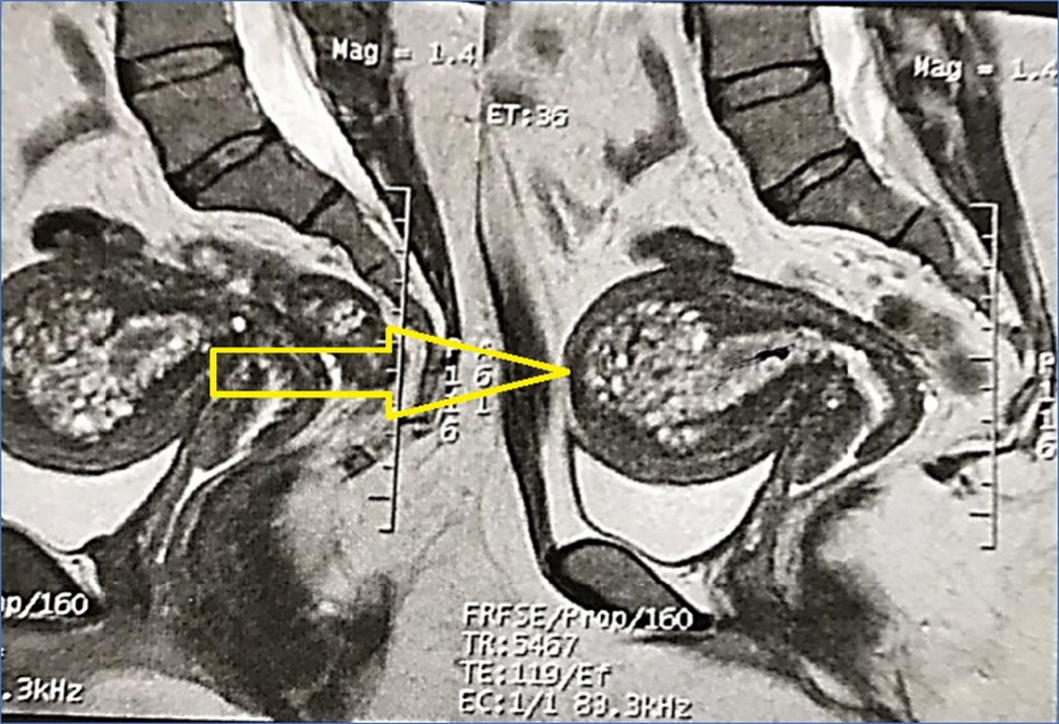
MRI unveils adhesions and uterine mass. MRI with sagittal section reveals an adhesion in the rectovesical space, along with adhesion of both ovaries to the uterus's body, suggesting a possible association with adenomyosis. A high‐signal multicystic, well‐defined mass is observed in the central part of the uterus on T2‐weighted sagittal images, measuring approximately 76 × 53 × 59 mm. The origin of the mass cannot be distinguished as either myometrial or endometrial. It extends near the serosal surface in the left fundal part. The serosal surfaces of the uterus are intact, and there is no evidence supporting the cervical invasion.
